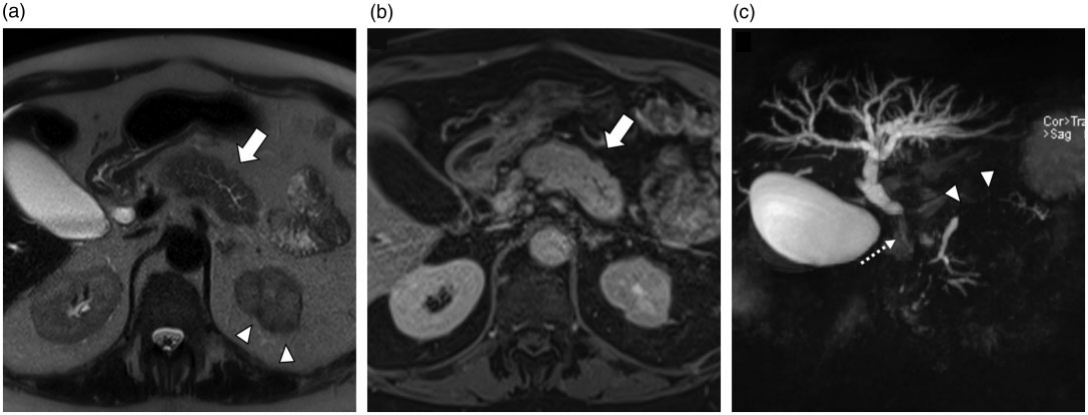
Magnetic resonance imaging (MRI) of a patient with autoimmune pancreatitis (AIP) type 1 and other organ involvement (OOI). The T2-weighted axial image (a) and the venous phase (b) show a diffuse enlargement of the pancreas (also known as ’sausage pancreas’) (arrows). The maximum intensity projection (MIP) reformatted image of the magnetic resonance cholangiopancreatography (MRCP) sequence shows a long stricture of the main pancreatic duct (MPD) without upstream dilatation (arrowheads), and a stricture of the distal common bile duct (CBD) with upstream dilatation (dotted thin arrow). Furthermore, there are hypointense cortical lesions in the left kidney (a) (arrowheads) and worse contrast-enhancement in the renal parenchyma in the venous phase (b).
